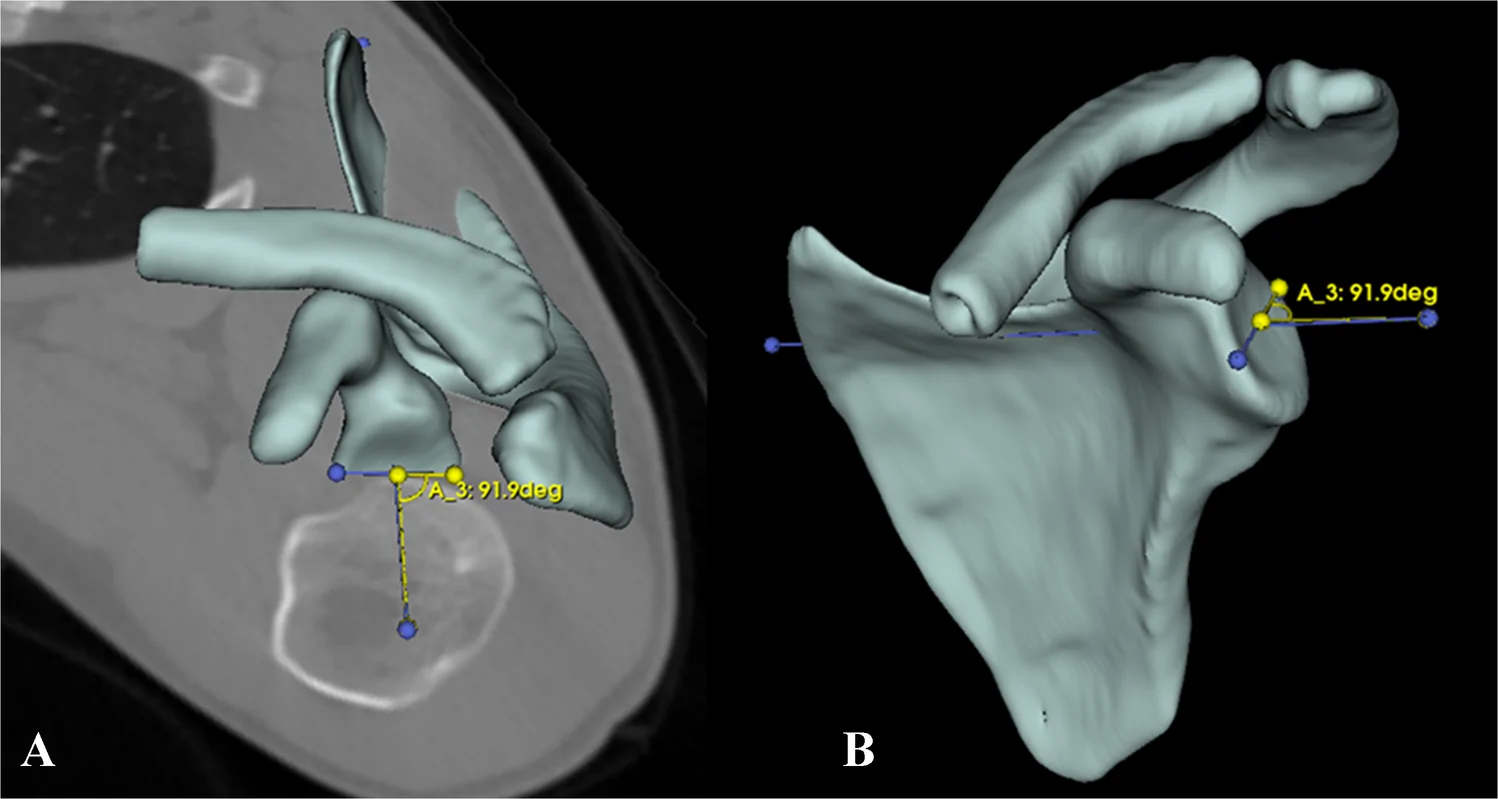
View of the glenoid version (GV) measurement on 3D-CT. A View from superior. B View from anterior
